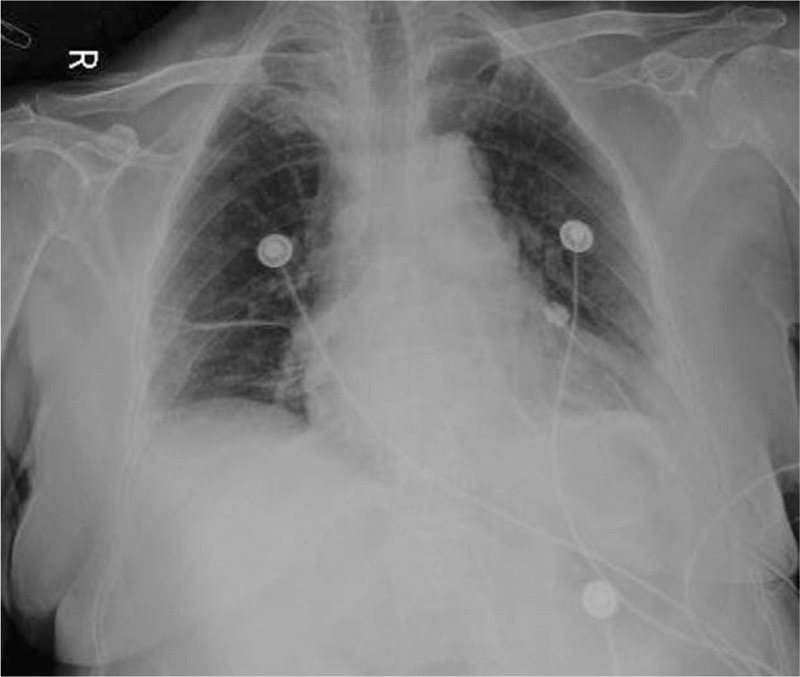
The first postoperative chest X-ray, performed 4 days after the operation.
